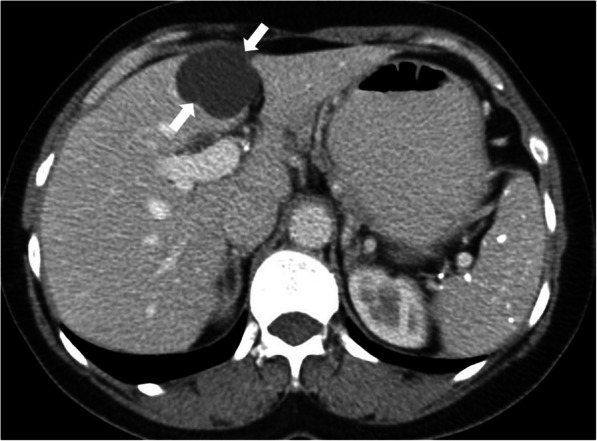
A 40-year-old female patient with known breast cancer. Axial plane post-contrast CT image shows a unilocular cystic lesion located in the subcapsular area of the segment 4 (arrows). The location was found to be typical for CHFC. The cyst was stable on 2-year follow-up study (not shown)
